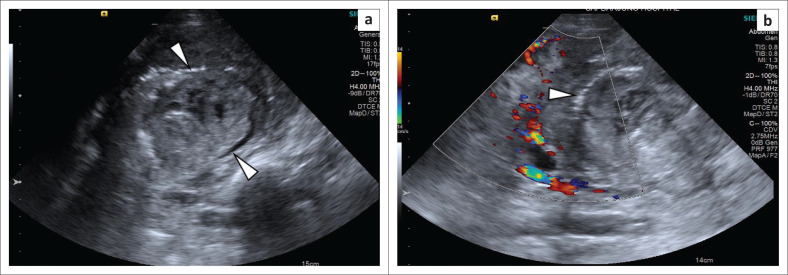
Grey scale (a) and Colour Doppler ultrasound (b) of the left kidney shows a large hetero-echoic left renal mass with intense vascularity at the periphery of the mass and a central avascular component (white arrow heads).
